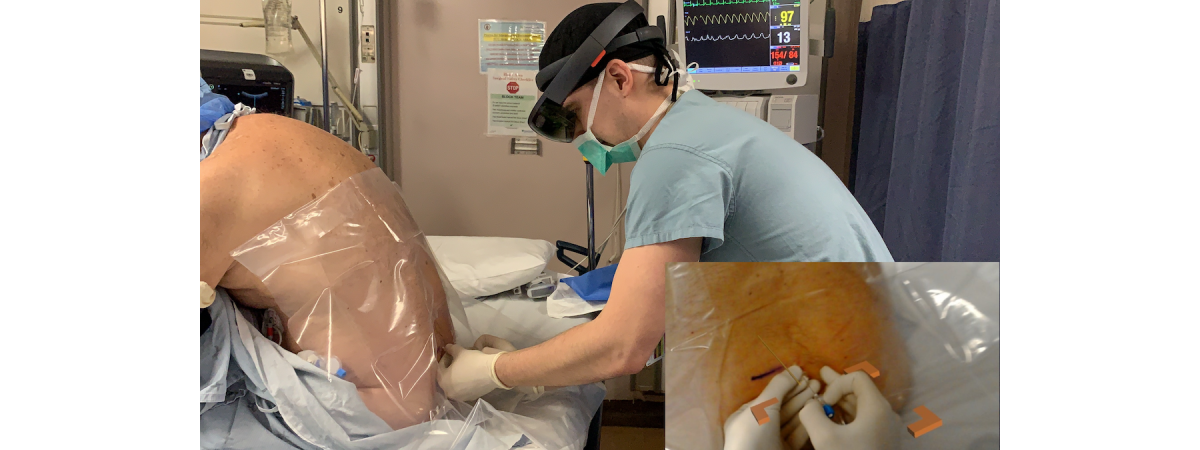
The user performs a neuraxial technique in standard sterile fashion. The inset shows the hologram projected into the user's visual field to aid replication of the ideal needle path as identified on ultrasound.
